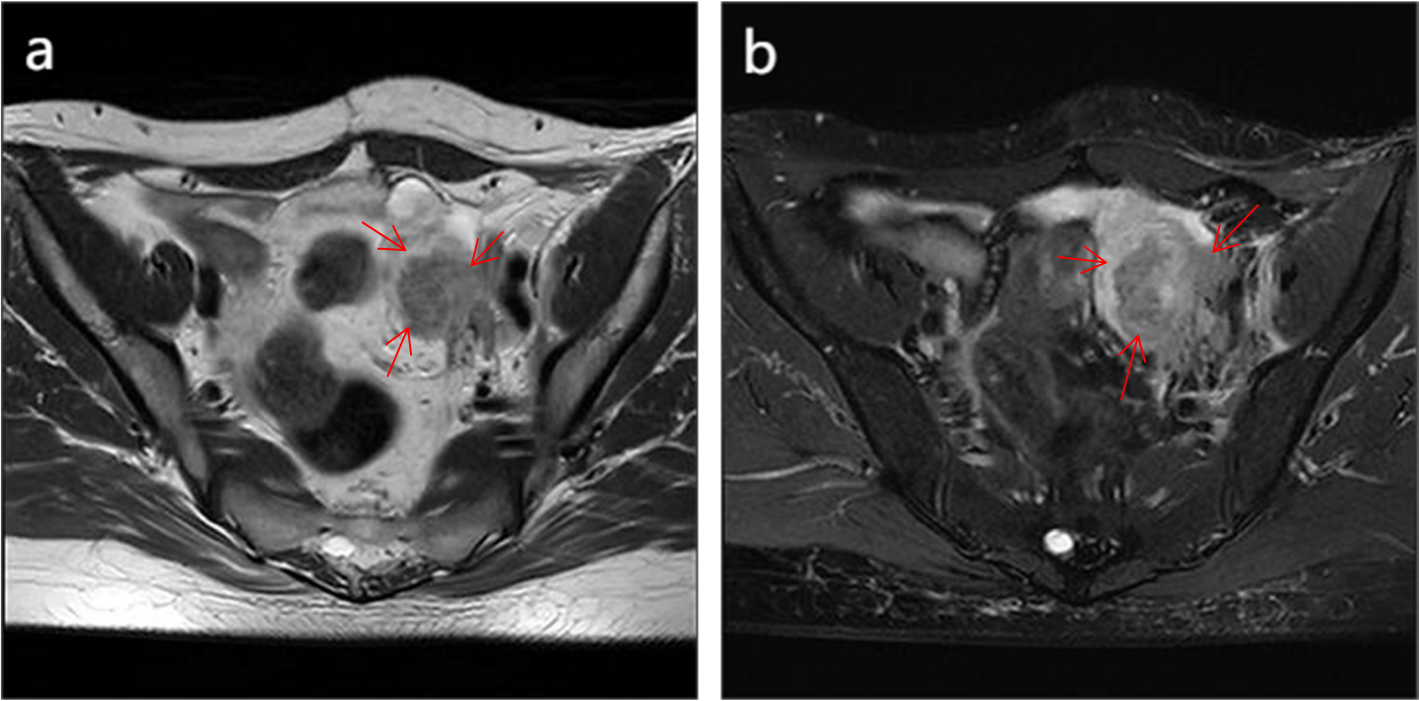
Pelvic magnetic resonance imaging demonstrating a pelvic solid tumor with marked enhancement. On T1-weighted magnetic resonance imaging, the tumor showed hypointensity. After administration of gadolinium-DTPA, the delayed phased image showed heterogenous tumor enhancement (arrow indicates pelvic tumor)
